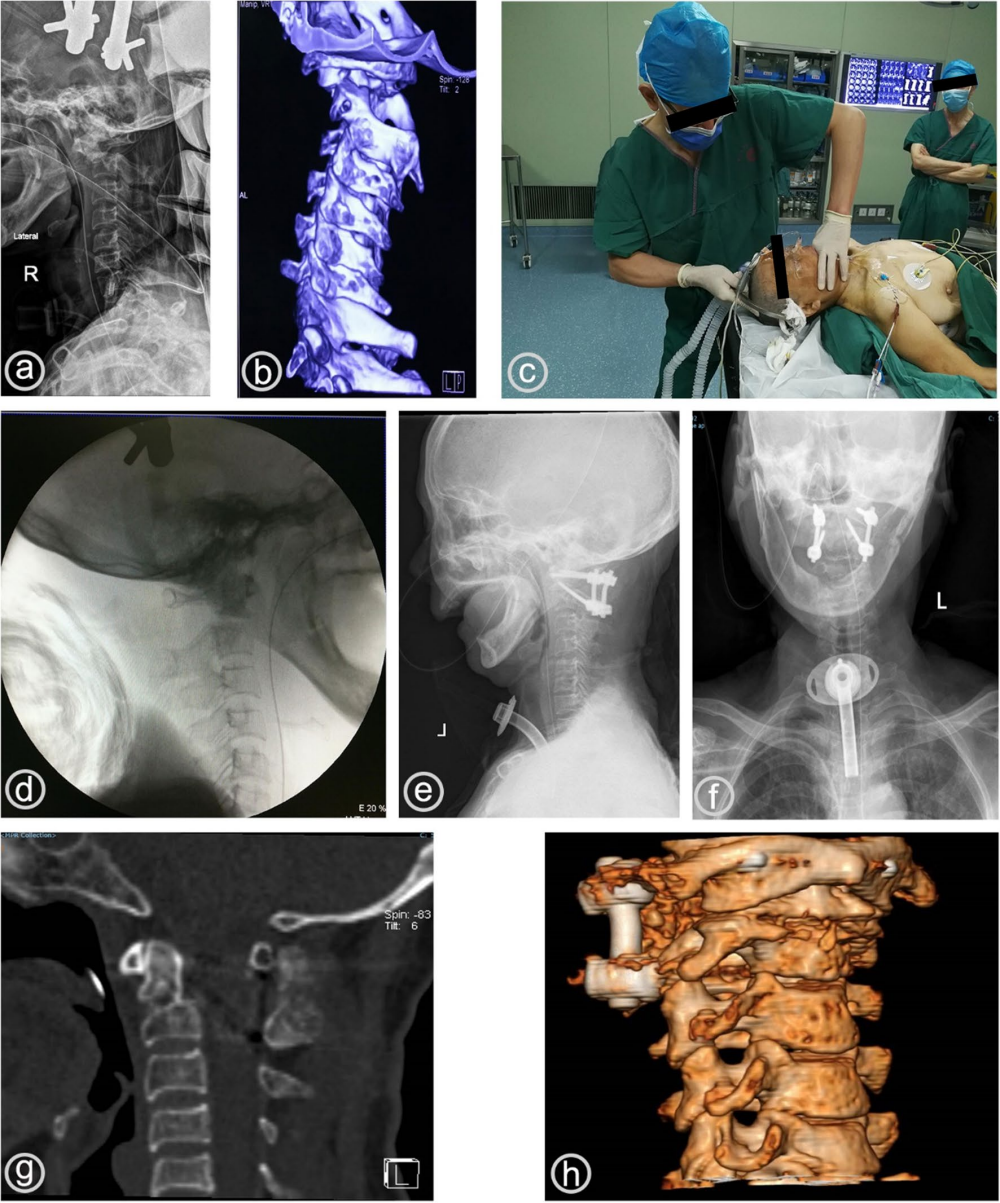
A bedside lateral radiograph 20 days after traction showing atlantoaxial subluxation and interlocking (a); 3D Computed tomography (CT) before reduction showing atlantoaxial subluxation with lateral block joint interlocking (b); closed reduction via the oropharynx after general anesthesia (c); intraoperative fluoroscopy after reduction showing release of atlantoaxial interlocking and correction of subluxation (d); postoperative review of anterior and lateral radiographs showing good internal fixation (e, f); CT sagittal view and 3D reconstruction showing atlantoaxial subluxation. The spinal cord compression was released and the atlantoaxial spine was fused with posterior implants (g, h)
